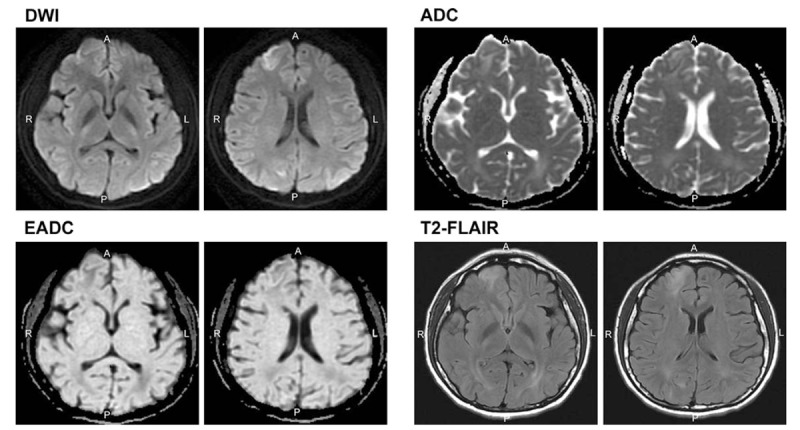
Persistent high-signal intensity lesions on diffusion-weighted imaging (DWI), T2 fluid-attenuated inversion recovery (FLAIR) in the right frontal and parietal lobes. Decreased signal intensities are observed on apparent diffusion coefficient (ADC) maps in the right temporo-occipital and splenium of the corpus callosum, suggesting a partial reversal of vasogenic edema. Total reversal of cytotoxic edema is observed in the splenium of the corpus callosum.
